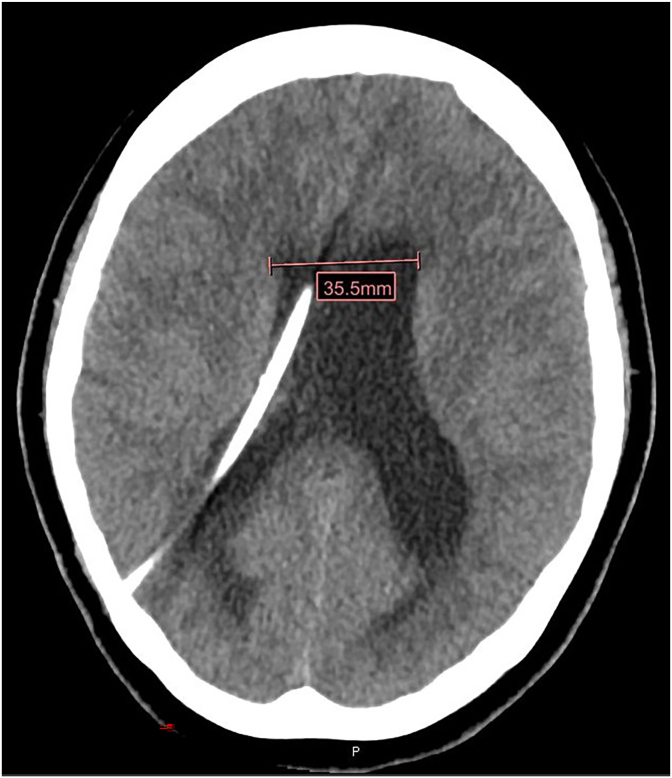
Computed tomography of the head on Day 0 shows mild to moderate hydrocephalus with the presence of a right posterior parietal VP shunt tube that traverses the right lateral ventricle.
